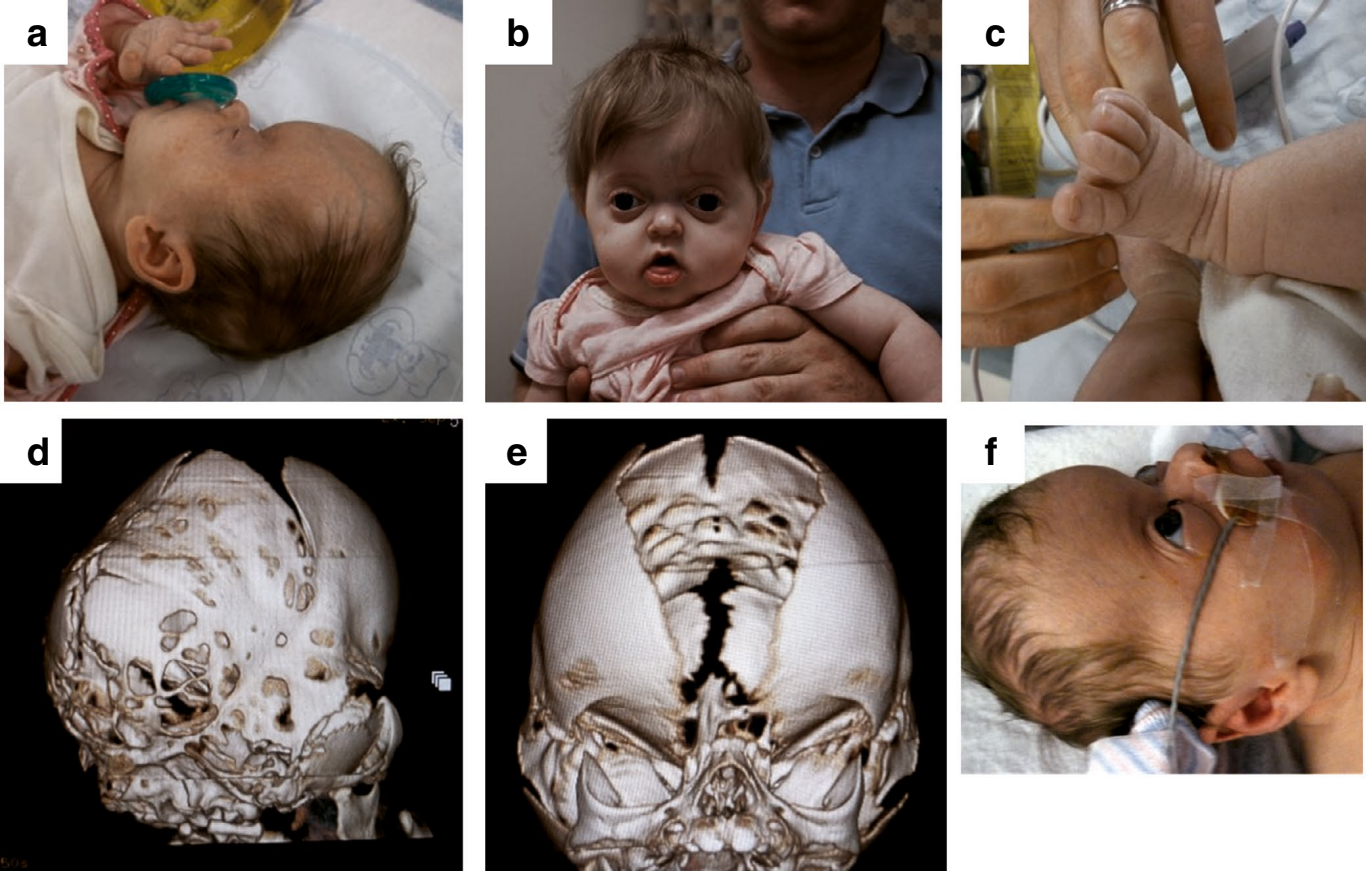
Clinical findings of CS‐39. (a) Severe turribrachycephaly and flattened lower occiput are evident. (b) Midface hypoplasia, beaked nose, and (c) broad toes are observed. (d, e) CT scans show lambdoid, metopic and bicoronal suture fusion. (f) Severe bilateral exorbitism and low set ears are observed
